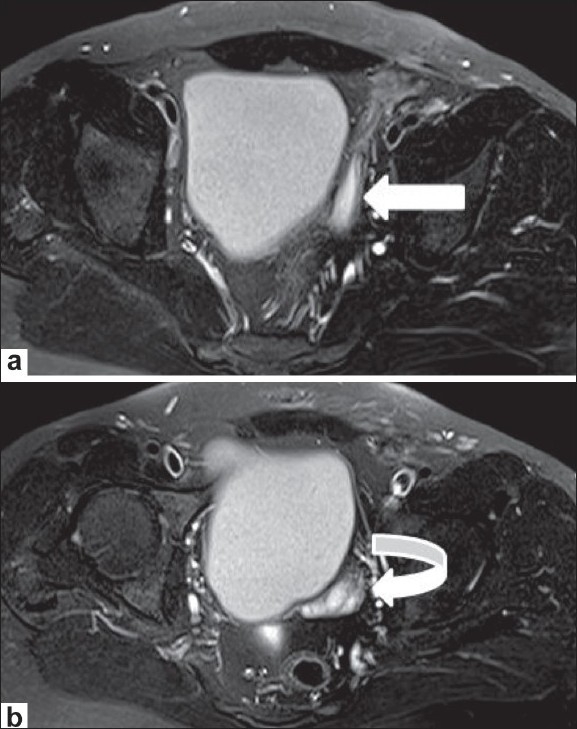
Axial T2W MRI images (a and b) at the level of the acetabulum show a vagina-like structure (arrow in a) extending toward the left seminal vesicle (curved arrow in b) in the next axial section
